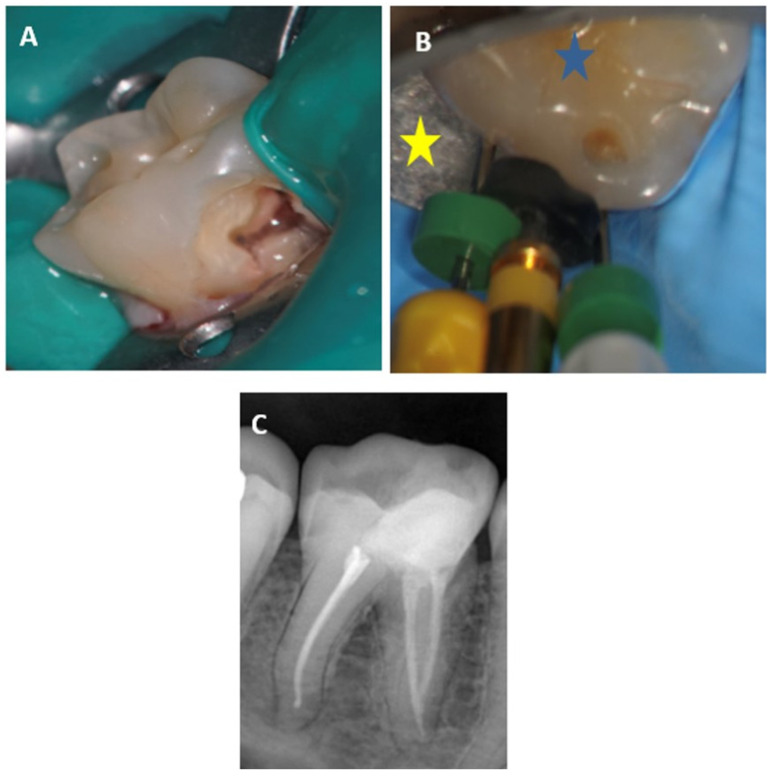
Mandibular molar with a buccal access cavity: (A)—the access cavity after the pulp was exposed; (B)—the files are inserted into the canal *(blue star*—the occlusal plane of the tooth; *yellow star*—the buccal aspect of the dental clamp); (C)—final X-ray image of the root canal, after obturations with GP and TSS.
